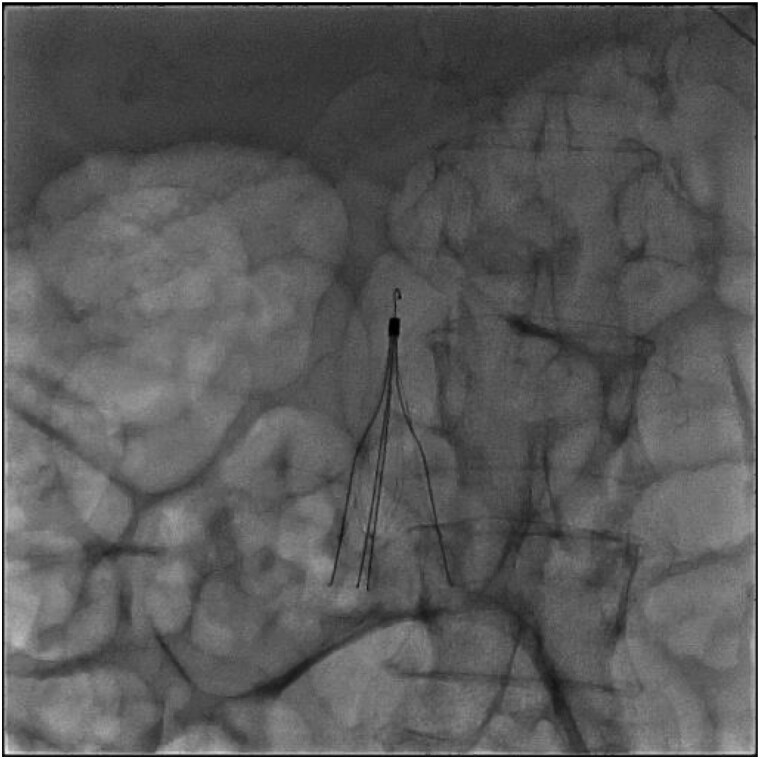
X-ray fluoroscopy showing placement of an inferior vena cava filter for the prevention of recurrent embolism.
